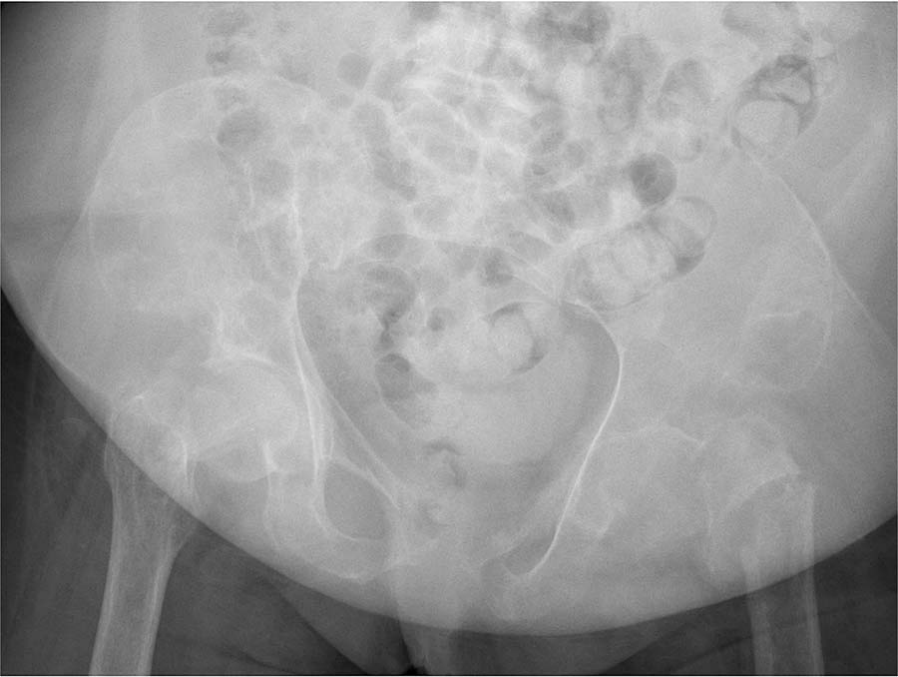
Pelvic x-ray coronal view, linear lucency through the left femoral neck with associated cortical irregularity and focal discontinuity of the medial and lateral cortex concerning for pathological fracture.
